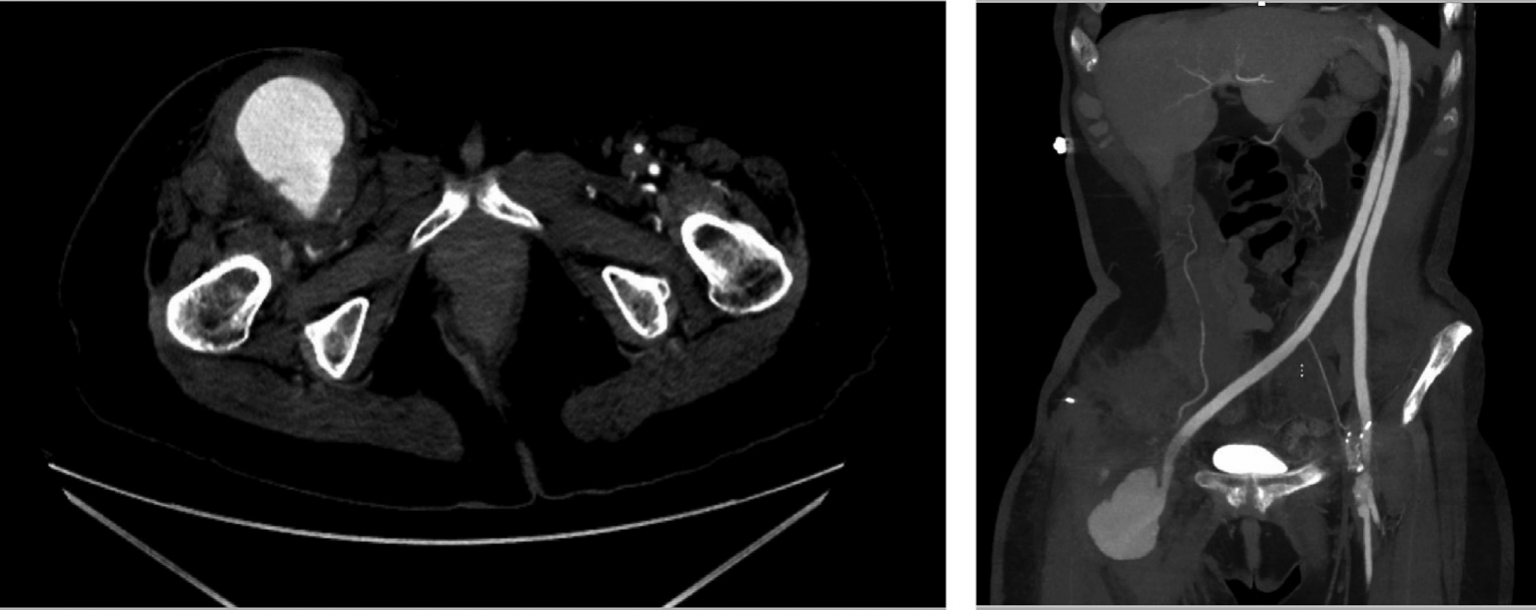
Computed tomography images of right femoral artery pseudoaneurysm (PSA).
